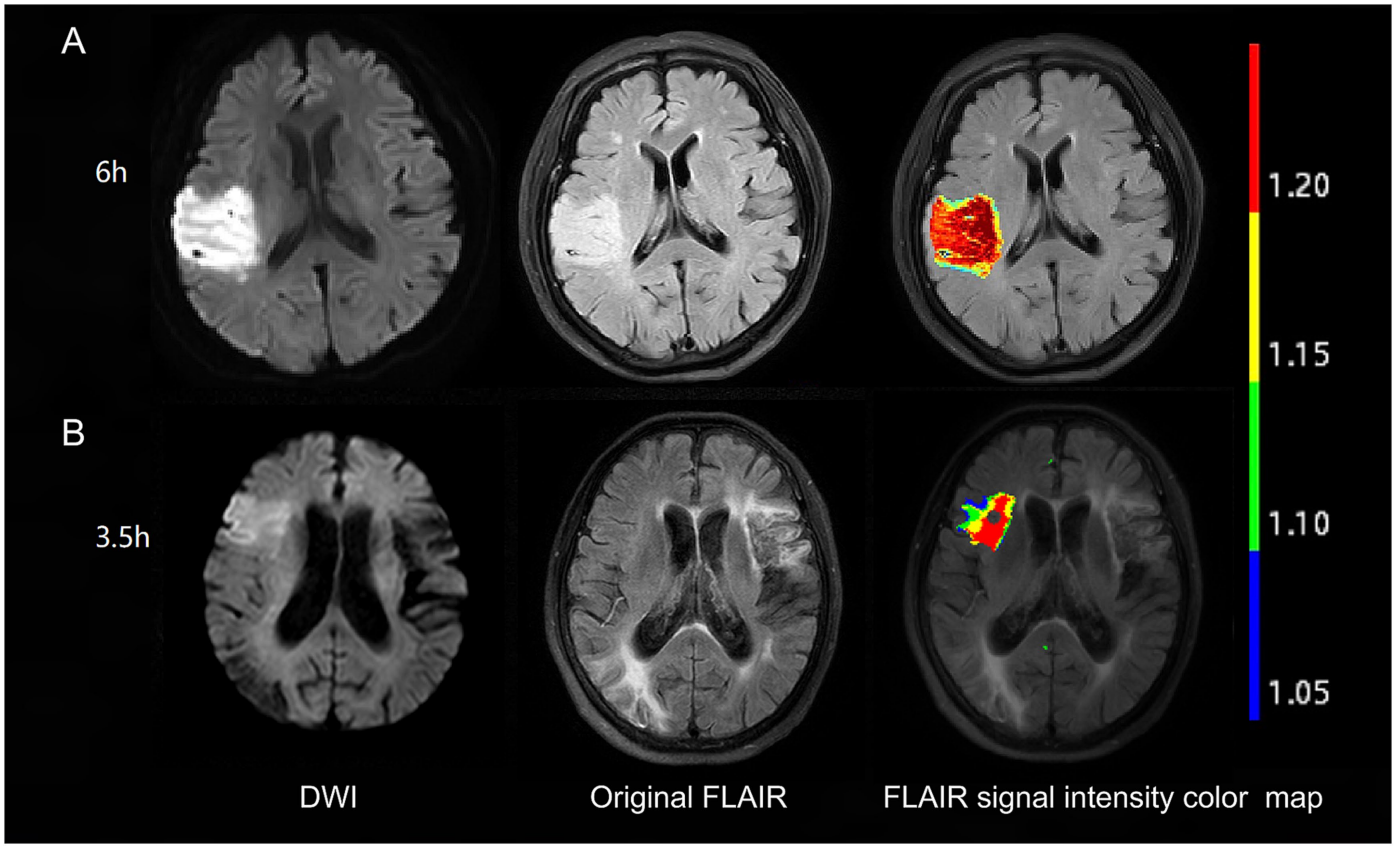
Examples of FLAIR signal intensity color maps in two patients. Patient with 6 h from symptom onset to MRI time (A) has no DWI-FLAIR mismatch with FLAIR SIR of 1.25. Patient with 3.5 h from symptom onset to MRI time (B) has DWI-FLAIR mismatch with FLAIR SIR of 1.13. FLAIR, fluid-attenuated inversion recovery; DWI, diffusion-weighted imaging; SIR, signal-intensity ratio.
